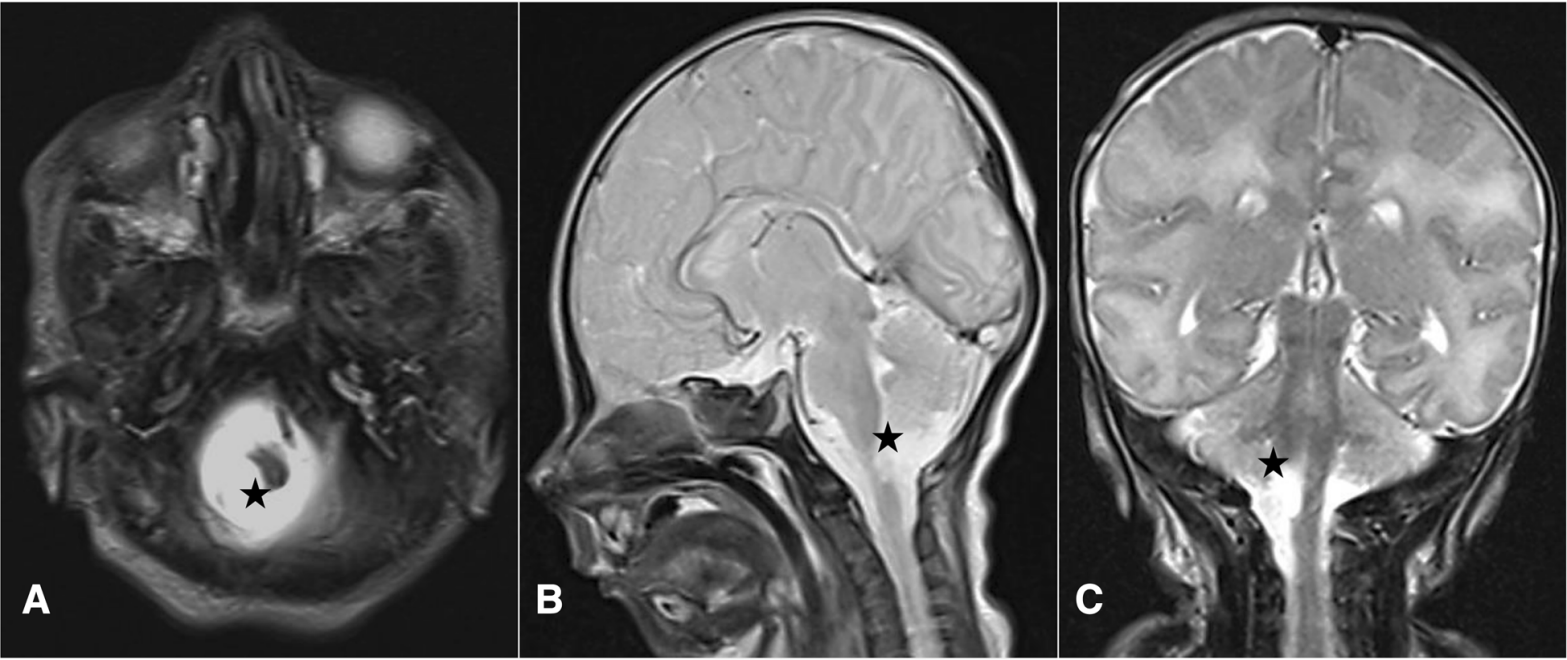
Cerebral MRI. Axial (a), Sagittal (b) and Coronal (c) T2-weighted sequence showing deformation of the cervicomedullary junction with a focal defect within the right medulla oblongata (black stars)
